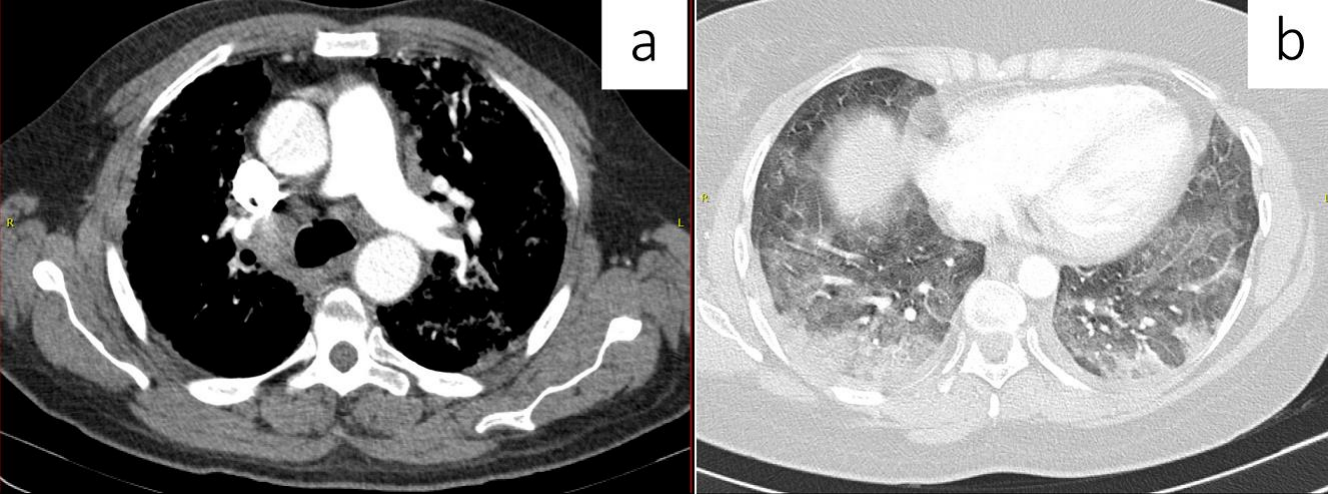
a) axial CT soft tissue window demonstrating mediastinal lymphadenopathy; b) axial CT lung window demonstrating bilateral pleural effusions with mixed ground glass and reticular changes
